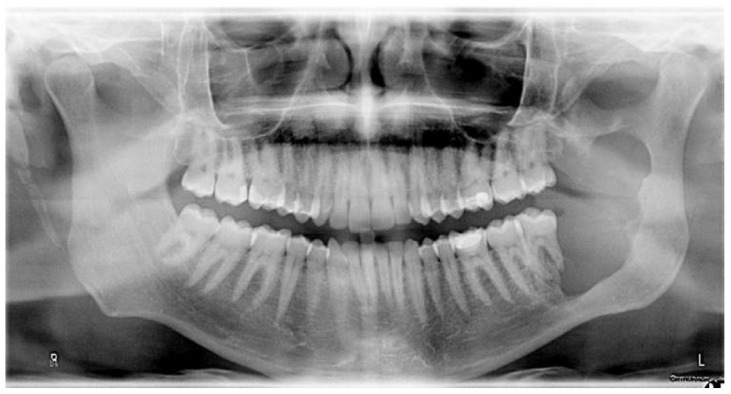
Preoperative orthopantomogram. A well-defined, scalloped radiolucent area is visible in the left mandible, extending towards the coronoid process. Teeth 37 and 38 are involved in the lesion.
